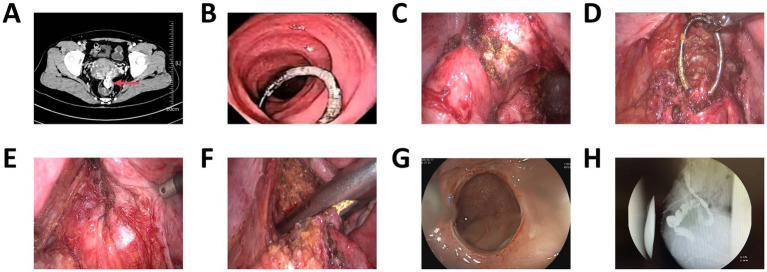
Clinical, intraoperative and postoperative imaging data of the patient with IUD-related complex high rectovaginal fistula. (A) Preoperative pelvic contrast-enhanced computed tomography, with an arrow pointing to the migrated metallic IUD embedded in the rectal wall. (B) Preoperative colonoscopy showing the annular metallic IUD protruding into the rectal lumen. (C) Intraoperative exploration showing the rectovaginal fistula tract at the posterior vaginal fornix. (D) Intraoperative photograph of the completely removed oval metallic IUD. (E) Intraoperative photograph showing tension-free suturing of the posterior vaginal wall fistula. (F) Intraoperative photograph of the resected rectal segment containing the fistula tract and IUD embedding site. (G) Postoperative colonoscopy at 3 months, showing well-healed rectal anastomosis with no residual fistula. (H) Postoperative defecography at 3 months, confirming no fistula recurrence and normal rectoanal functional morphology.
